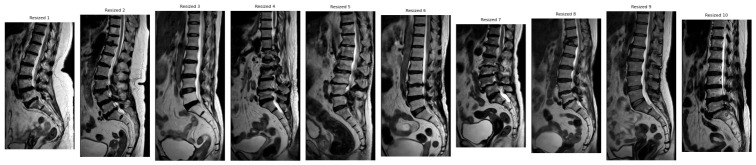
Representative preprocessed sagittal MR images after resizing and intensity normalization. Substantial variability in anatomy and image appearance highlights the need for robust localization strategies.
